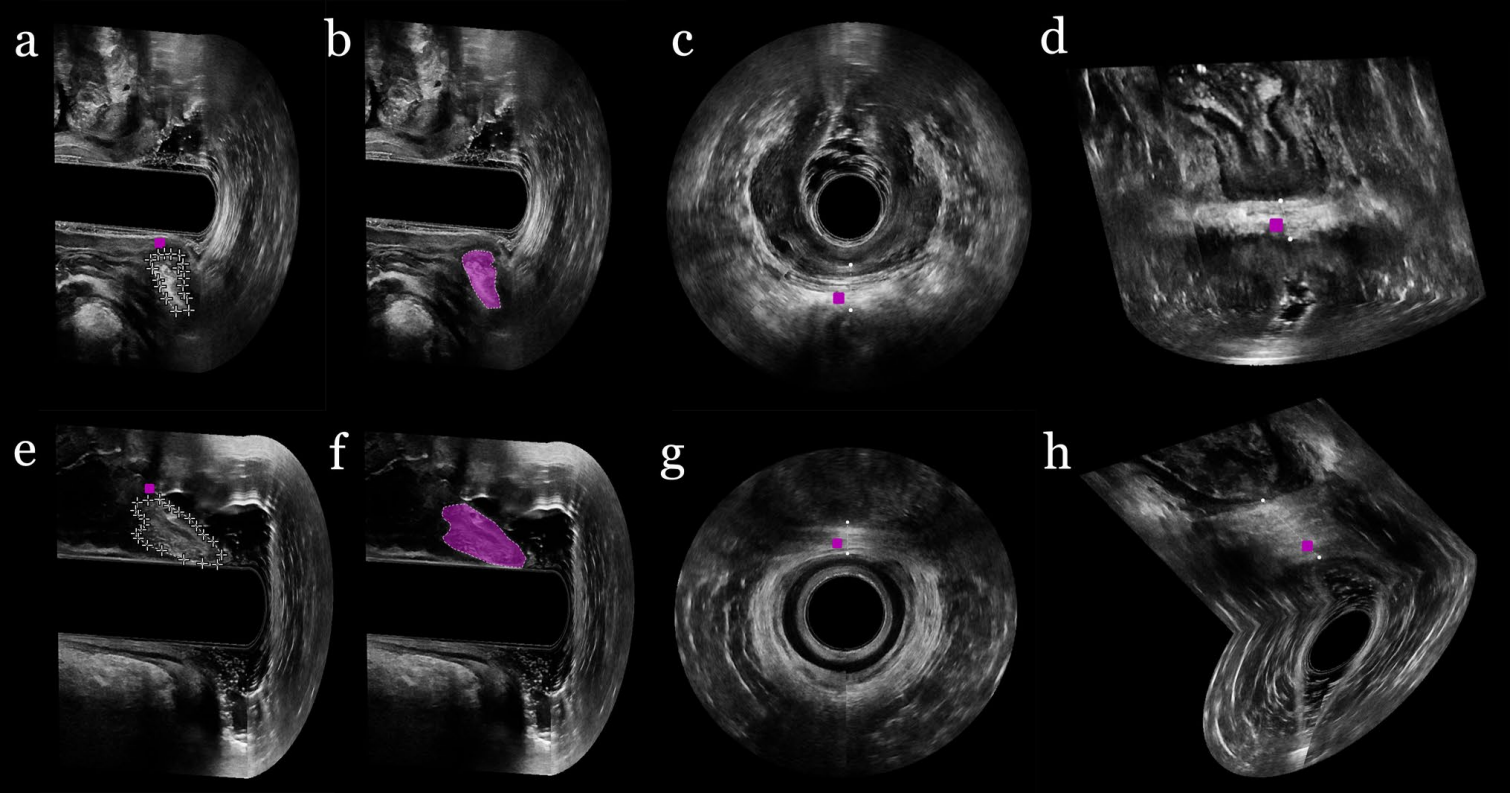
Three-dimensional ultrasound imaging of the perineal body. Three-dimensional endovaginal ultrasound: a Area in the sagittal plane; b Area in the sagittal plane, highlighted in pink; c The dotted line showing the height in the axial plane; d The dotted line showing the length in the coronal plane. Three-dimensional endoanal ultrasound: e Area in the sagittal plane; f Area in the sagittal plane, highlighted in pink; g The dotted line showing the height in the axial plane; h The dotted line showing the length in the coronal plane
